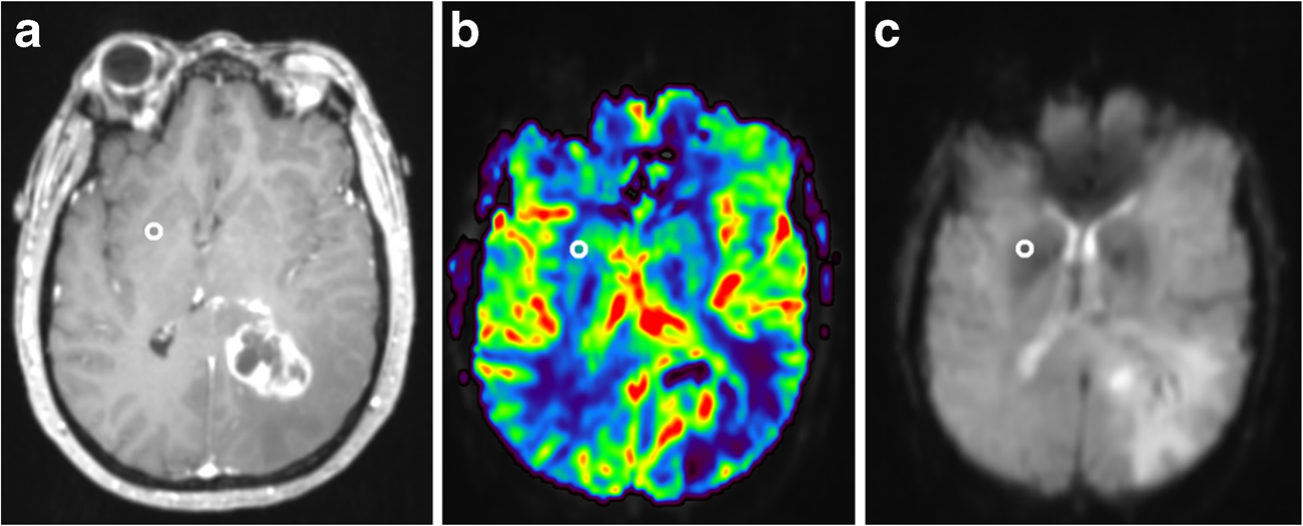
Example of distortion artifacts in the putamen in a patient with a glioblastoma in the left hemisphere (A contrast-enhanced T1-weighted MR image, B cerebral blood volume (CBV) map from dynamic susceptibility contrast-enhanced MRI (DSC-MRI), C source DSC-MR images). Note that the CBV map is calculated, also in the putamen area. However, DSC-MR source images show distortion artifacts in the putamen area. Despite these distortions rCBV could be calculated in the putamen area
